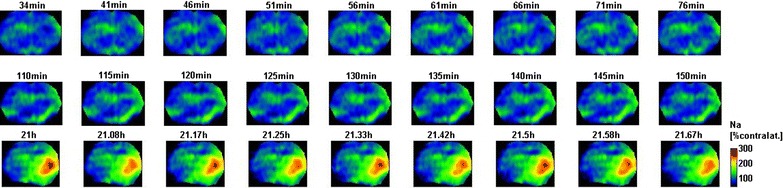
Representative example of sodium MR images acquired before (*first row*), after (*second row*), and 1 day after reperfusion (*third row*)
